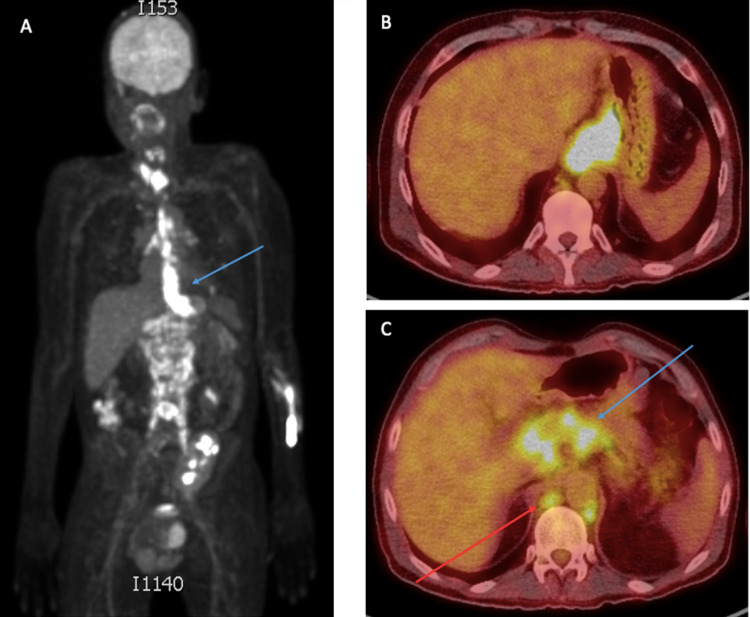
PET/CT whole body scan A) Coronal maximum intensity projection image of a whole-body 18F-FDG PET scan showing marked FDG-avid circumferential wall thickening at the mid and distal thirds of the esophagus extending to the gastric fundus corresponding to the known malignancy (blue arrow). B) Axial-fused 18F-FDG PET/CT image showing a marked FDG-avid lower esophageal mass corresponding to the known malignancy. C) Axial-fused 18F-FDG PET/CT image showing marked FDG-avid conglomerate gastrohepatic/celiac (blue arrow) and retrocrural (red arrow) lymphadenopathies consistent with metastasis.
